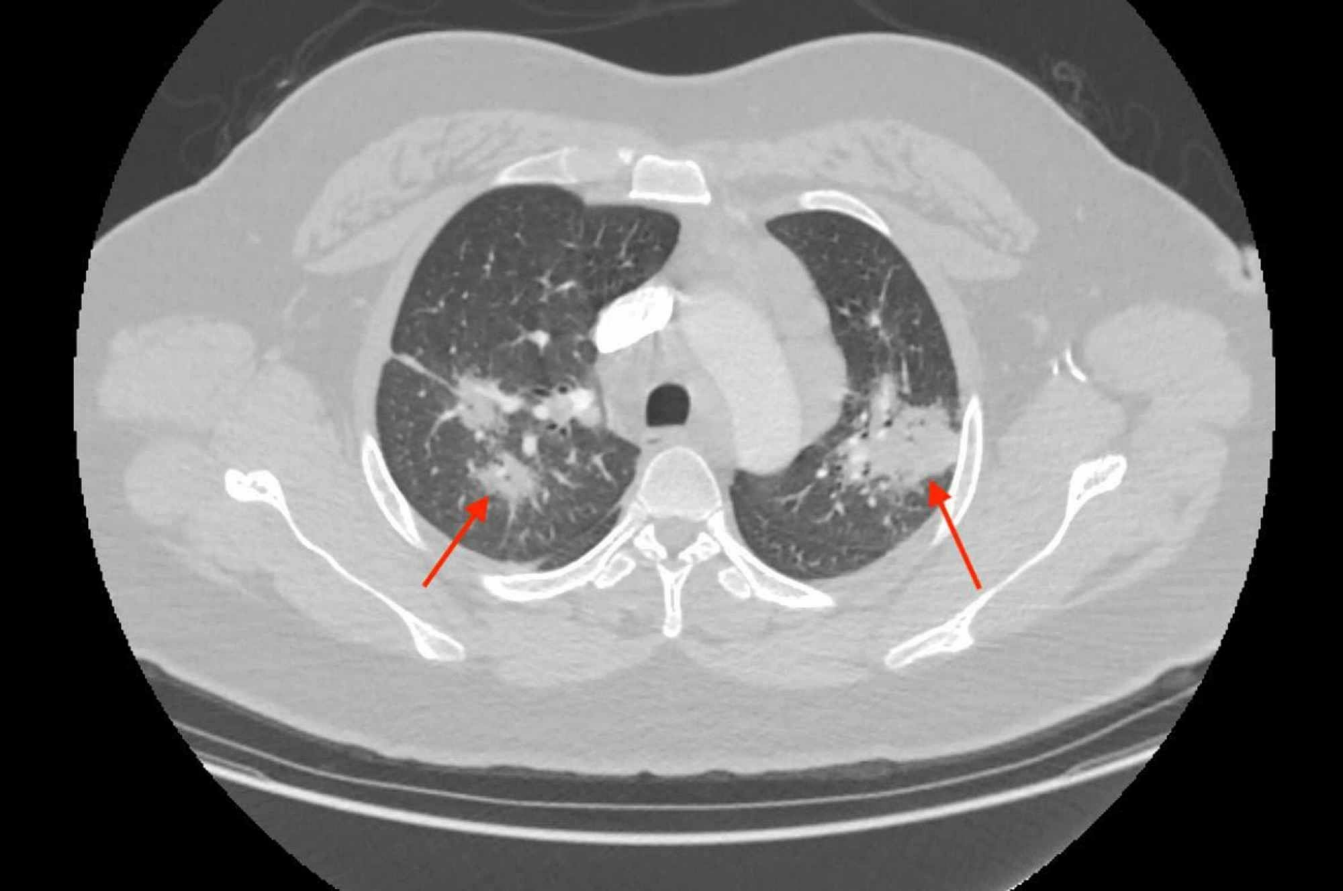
CT angiography chest transverse view showing bilateral pulmonary infiltrates.
